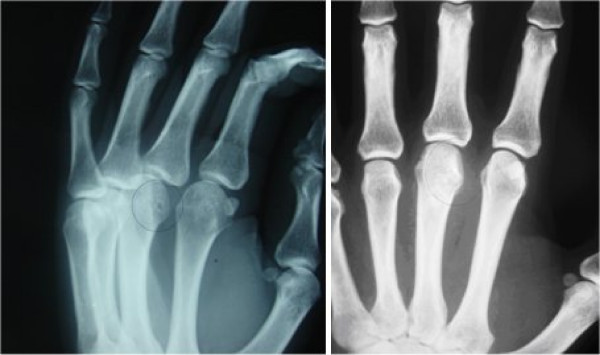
Plain radiography of the left hand. A small, oval, radiolucent lesion partially surrounded by sclerotic bone (left). No signs of recurrence at the 2-year follow-up (right).
